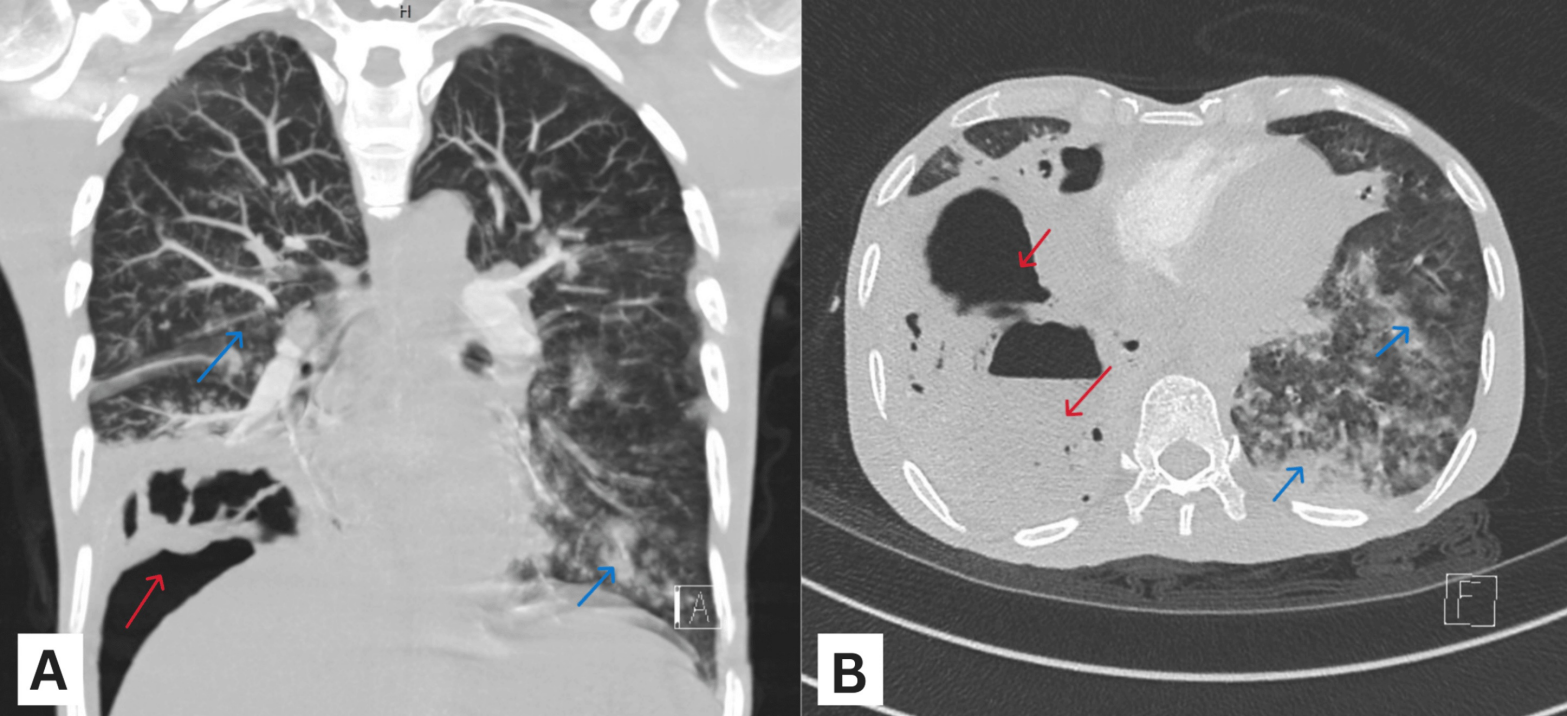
CT images showing the coronal view (A) and axial view (B) of a loculated cavitary consolidation with air fluid level in the lower lobe of the right lung (red arrows). Bilateral patchy infiltrates can also be seen (blue arrows). CT: computed tomography.
